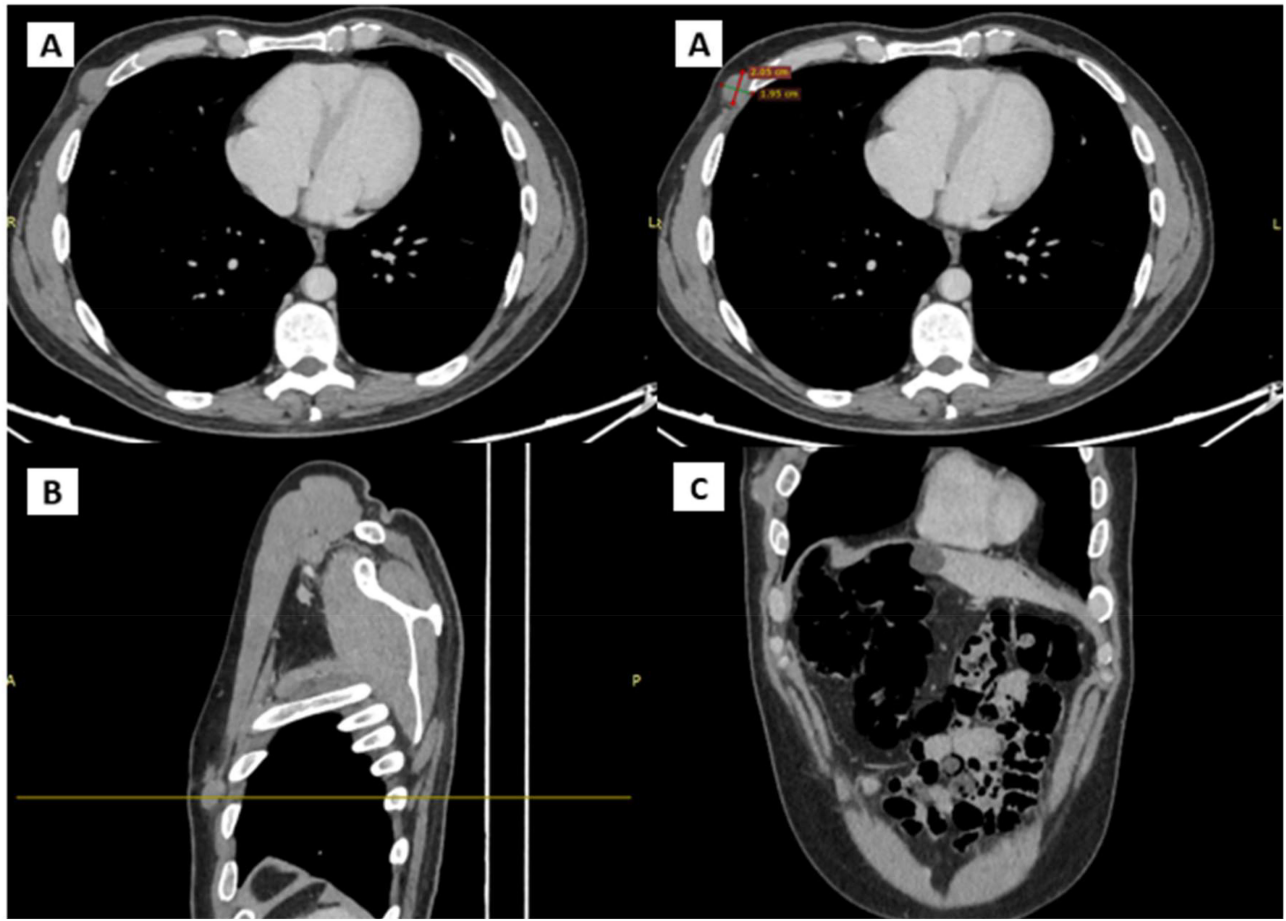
CT scans showing the presence and anatomical relation of the heteroplastic mass. (A) Transverse plane (B) Sagittal plane (C) Coronal plane.
